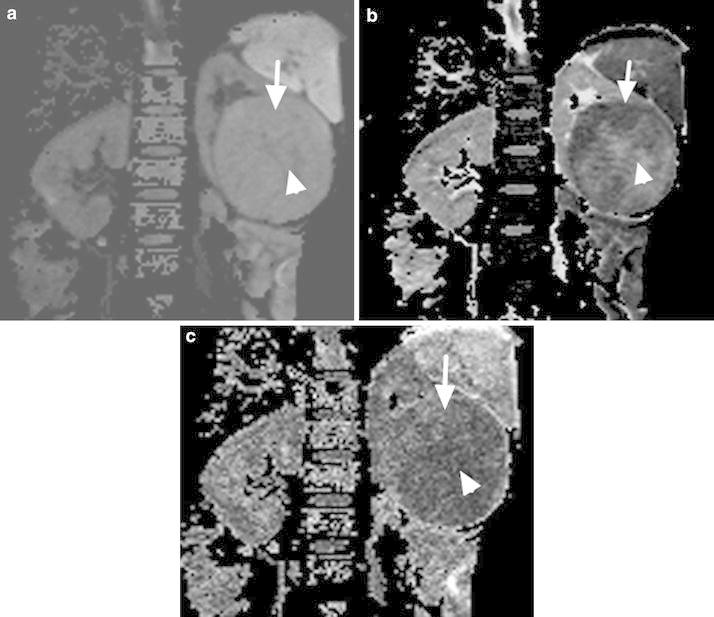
a–c. Images in 47-year-old man with CCRCC. a DWI map the solid parts of CCRCC is higher signal (*arrow*), the necrosis parts show low signal (*arrow head*), b ADC map the solid parts of CCRCC is lower signal (*arrow*), the necrosis parts show high signal (*arrow head*). c FA map similar to the ADC map
